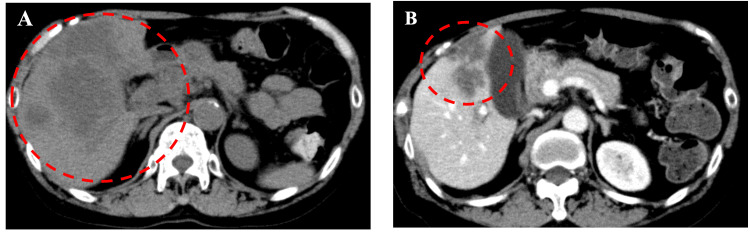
CT images demonstrating the initial response to combination therapy with encorafenib, binimetinib, and cetuximab (A) CT scan showing progression of the primary tumor after two cycles of treatment with durvalumab, gemcitabine, and cisplatin. (B) CT scan showing marked tumor shrinkage following two cycles of treatment with encorafenib, binimetinib, and cetuximab.
